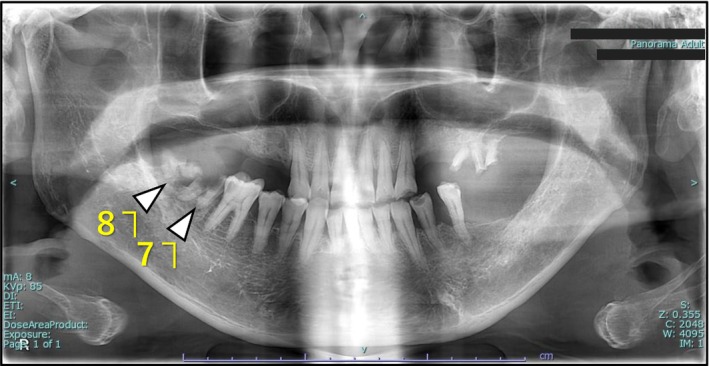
The preoperative orthopantomogram indicates the disrupted second and third molars in the right mandible which were the cause of the apical periodontitis (white arrows).
